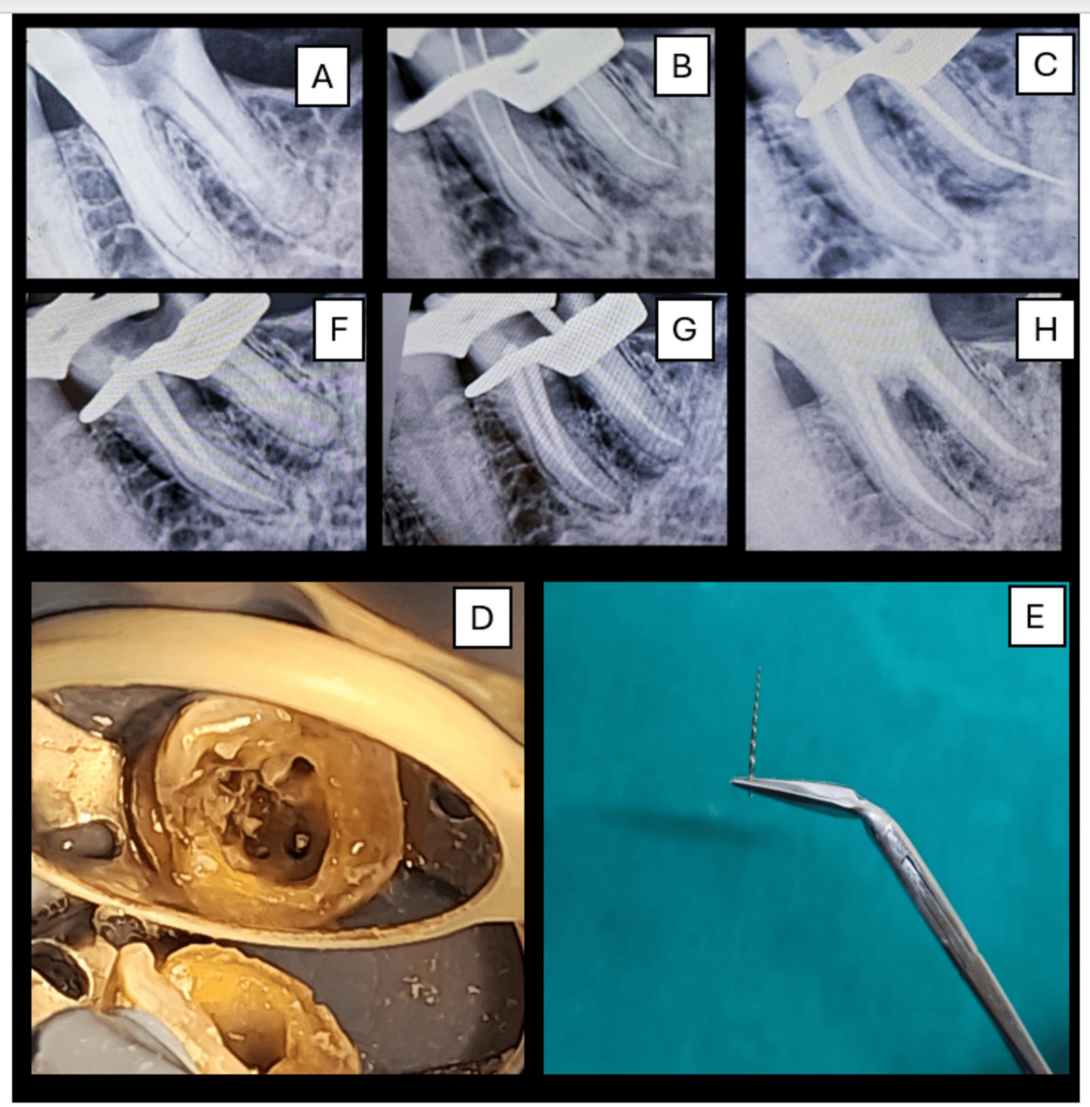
File retrieval from tooth 46 using ultrasonics. (A) Pre-op radiograph showing deep caries in #46 with periapical radiolucency. (B) Working length determination. (C) Separated ProTaper Gold file due to misjudged working length in the distal canal. (D) Ultrasonic agitation with the E15 tip to loosen the fragment. (E) Retrieved file fragment using Steiglitz forceps. (F) Radiograph confirming complete file retrieval. (G) Post-obturation radiograph. (H) Final radiograph showing furcal perforation repair with MTA and Abgel. MTA: mineral trioxide aggregate
